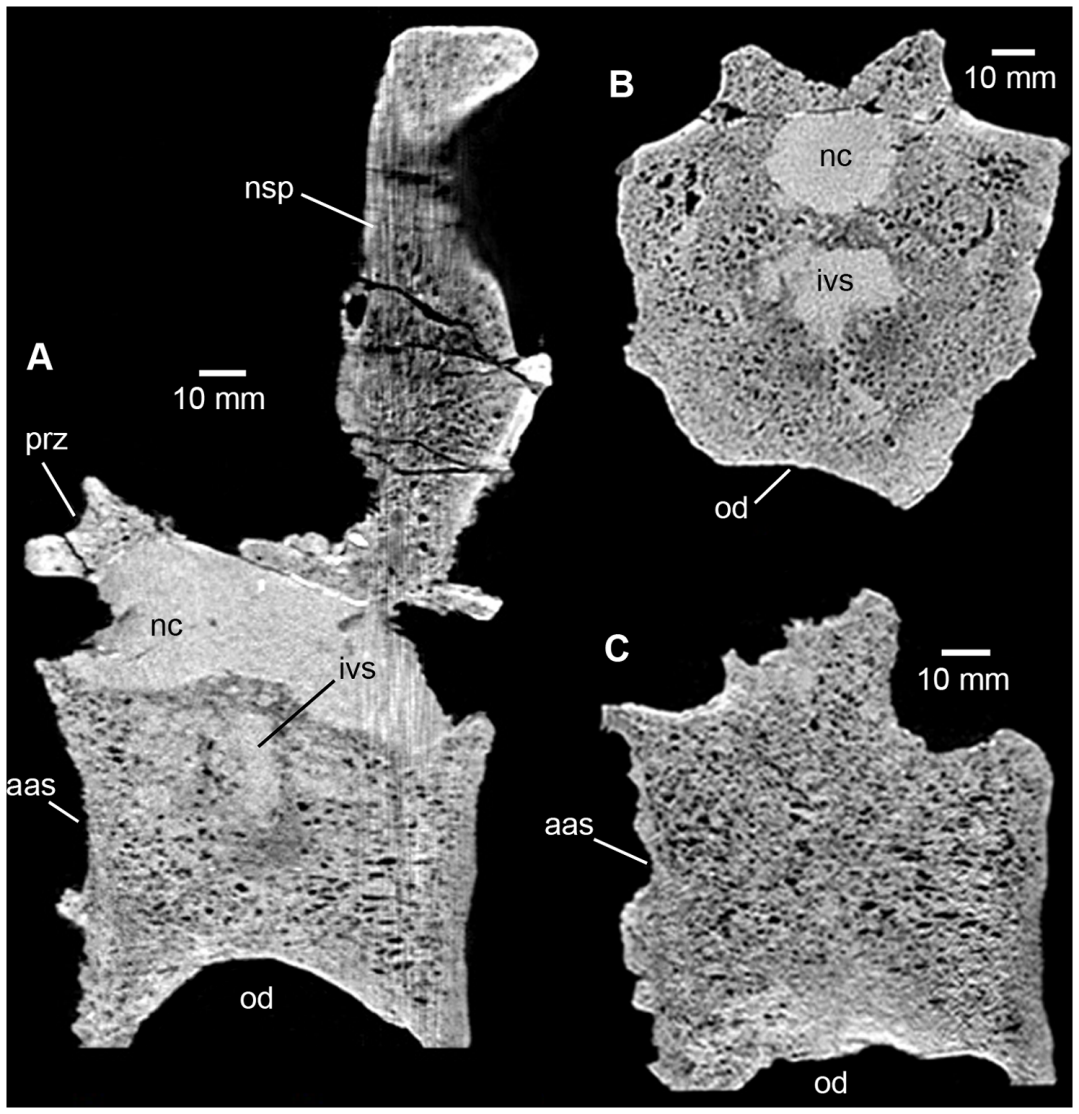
Phytosauria indet. from the Late Triassic of Halberstadt. CT scans of the fused vertebrae MB.R.1973. A. Sagittal section along the midline of the vertebrae. B. Transverse section through the intervertebral space, showing a simple cavity or lacuna. C. Sagittal section through the right lateral part of the vertebrae, showing their complete fusion. For the location of sections, please see Figure 6A, C. Abbreviations: aas, anterior articular surface; ivs, intervertebral space; nc, neural canal; nsp, neural spine; od, ovate depression; prz, prezygapophyses.
